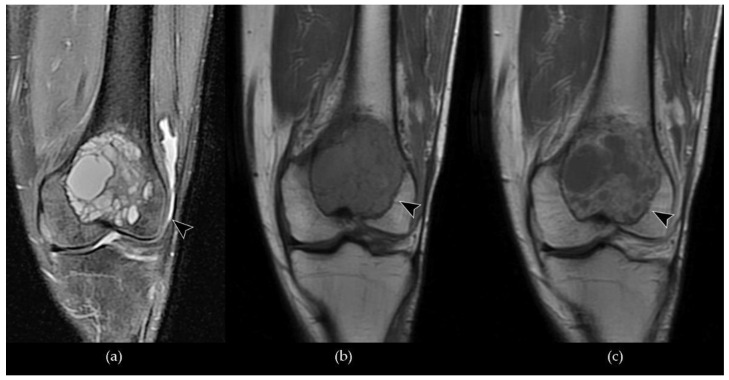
Coronal MRI of a GCTB of the distal metaepiphysis of the left femur (arrowhead) with associated joint effusion: (a) a fat-suppressed T2-W FSE scan shows a lesion with inhomogeneous signal hyperintensity in relation to the presence of multiple cystic components; (b) a T1-W FSE image shows non-uniform signal hypointensity; (c) a post-contrast T1-W FSE image demonstrates heterogeneous enhancement of the lesion, predominantly peripheral, and well discriminates the cystic and solid components.
